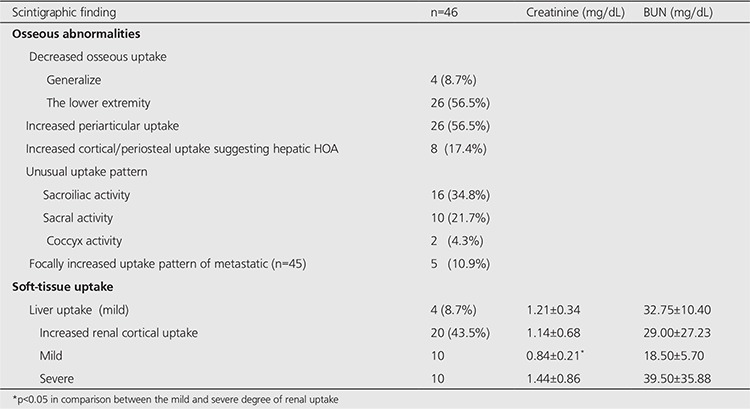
Osseous and nonosseous scintigraphic finding in adult LT candidates with end-stage CLD
